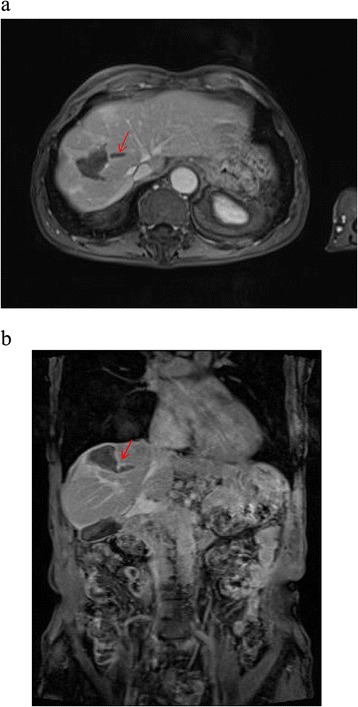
Axial (a) and coronal view (b) of MRI ViBE sequences after intra venous injection of contrast medium. In addition to the clear post-RFA coagulative necrosis, in the hepatic parenchyma it is visible the needle way, indicated by the arrow.
